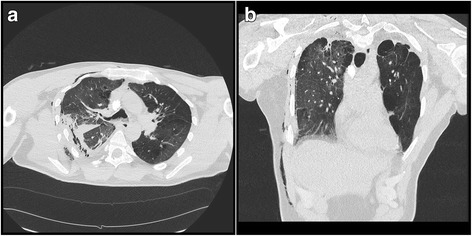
Preoperative computed tomography of the chest. Axial (a) and anterior (b) computed tomographic imaging indicating pulmonary contusion
